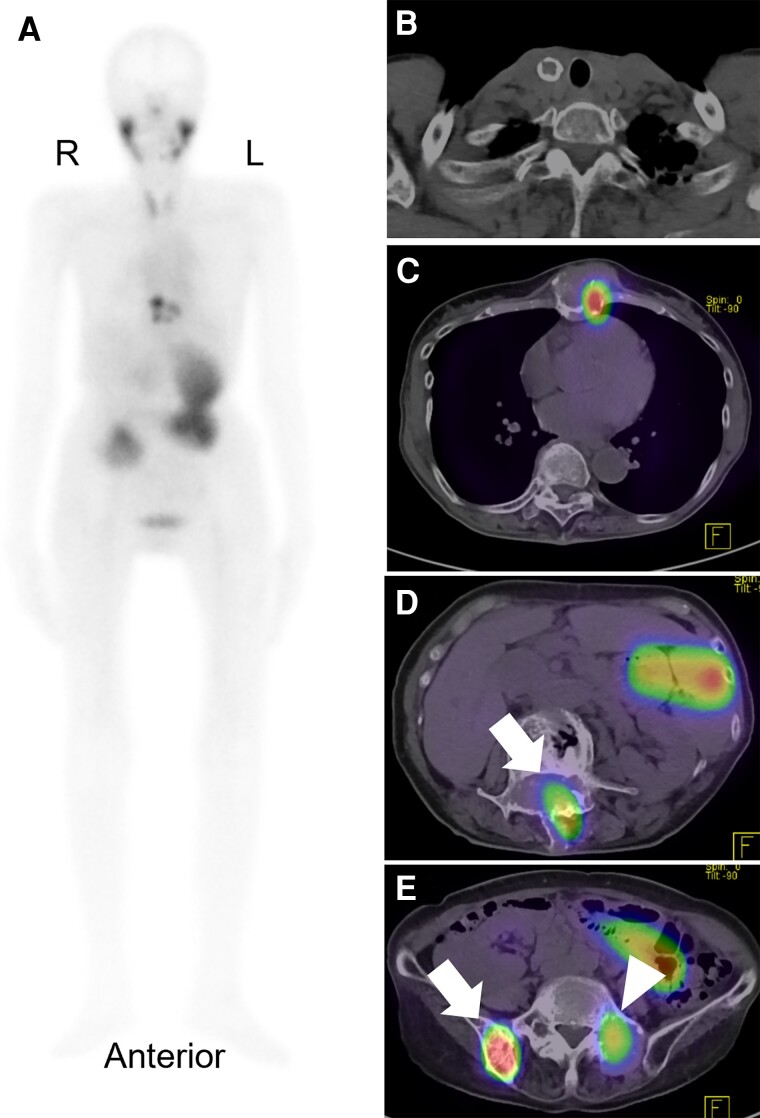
A, Whole-body ^99m^Tc-pertechnetate scintigram obtained at the recurrence of thyrotoxicosis. B, Although uptake at the thyroid bed was at the lower limit of normal, increased uptake is shown for the C, sternum; D, lumbar spine (white arrow); E, sacrum (white arrowhead); and E, right ilium (white arrow), despite decreased thyrotropin levels. L, left; R, right.
